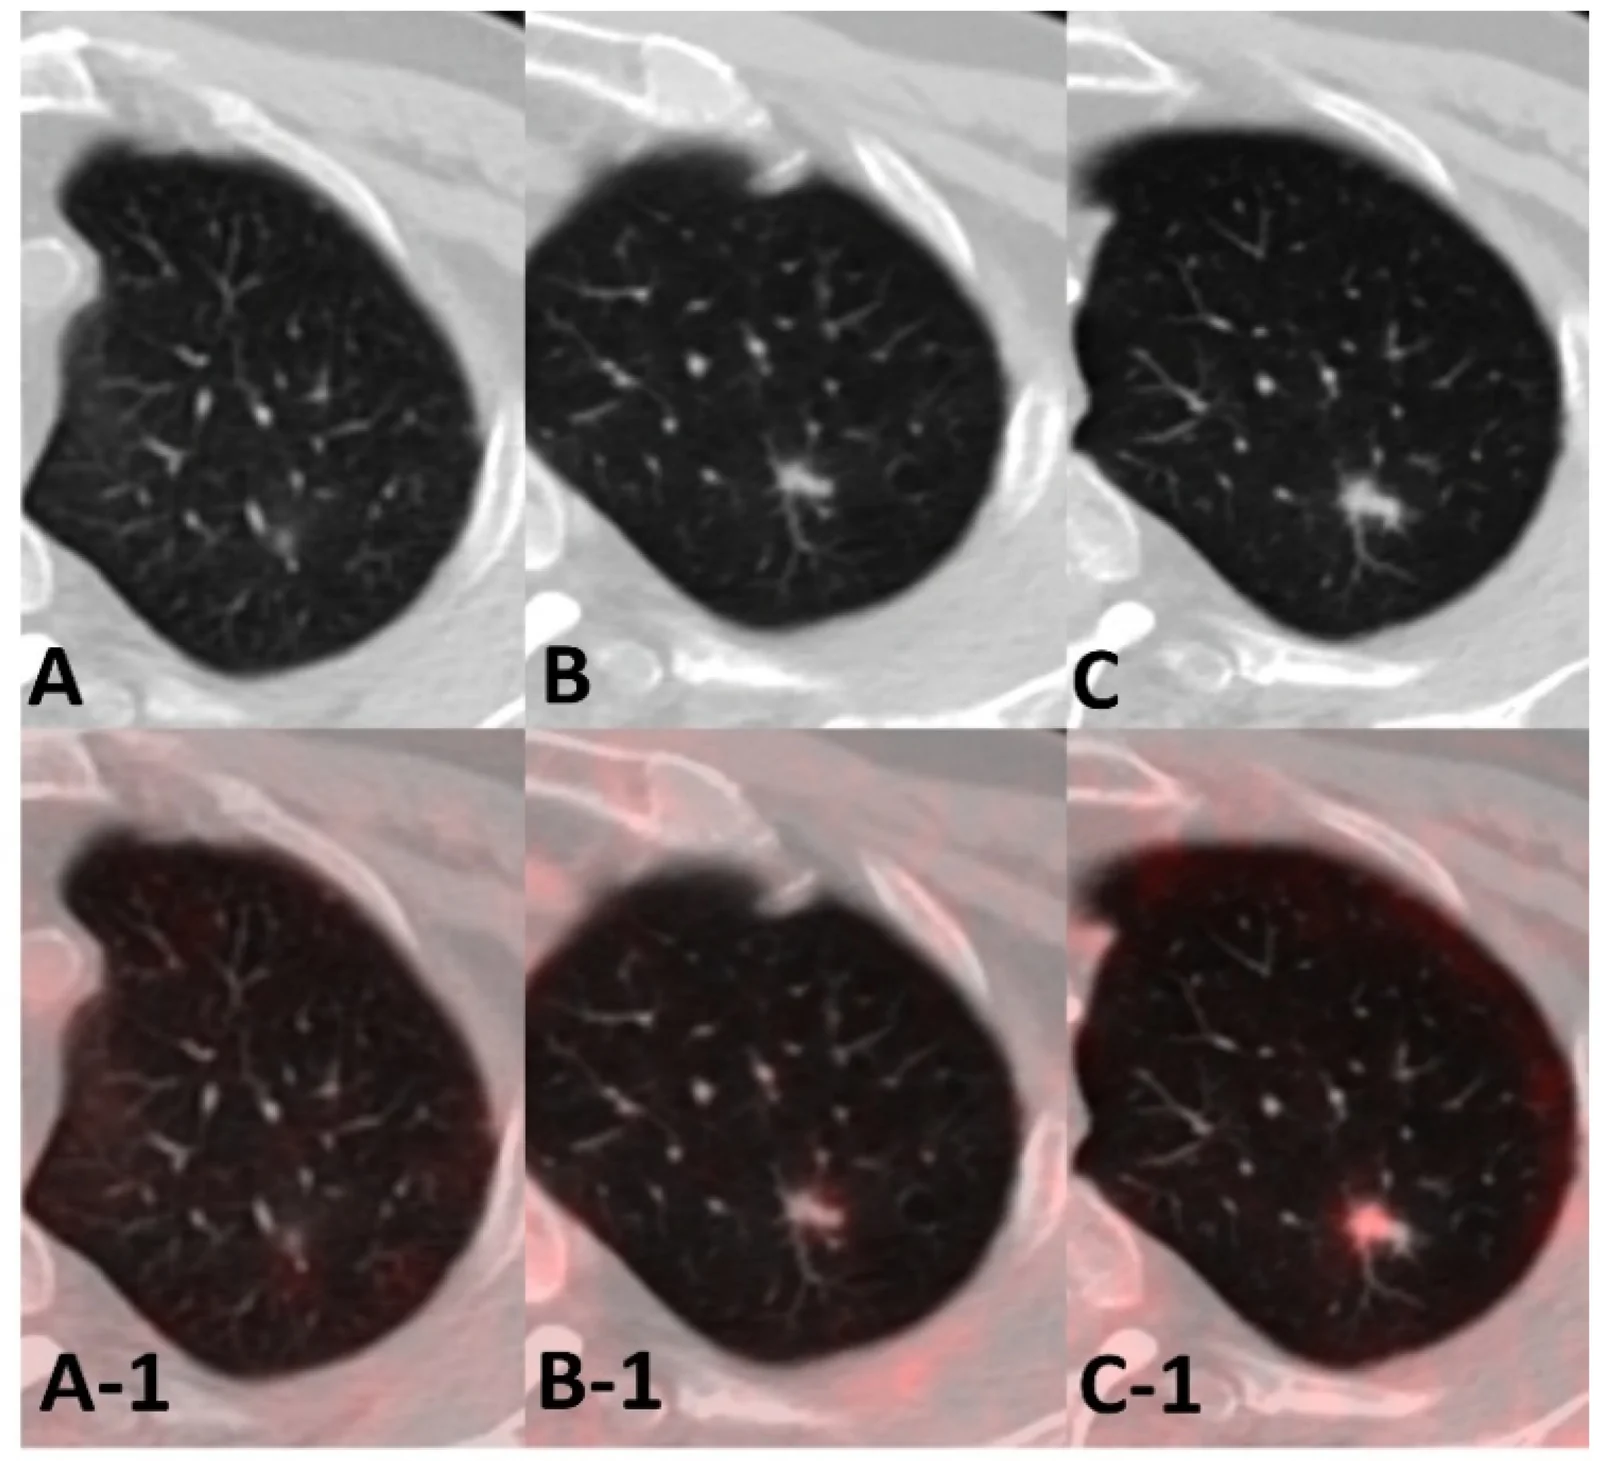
Subsolid nodule that proved to be a primary adenocarcinoma. A man under surveillance for lymphoma. (A,A-1) FDG PET/CT showed a small subsolid nodule in the left upper lobe with no discernible FDG uptake. (B,B-1) On follow-up after 2 years, a solid component and spiculated margins had developed, with only faint uptake (SUVmax 1.3). (C,C-1) After 4 years, focal uptake had become clearly visible (SUVmax 3.5) in the nodule, which had continued to enlarge. Left upper lobe bisegmentectomy revealed a moderately differentiated adenocarcinoma (acinar 60%, lepidic 40%; invasive component 0.6 cm of a 1.0 cm tumor, pT1aN0).
